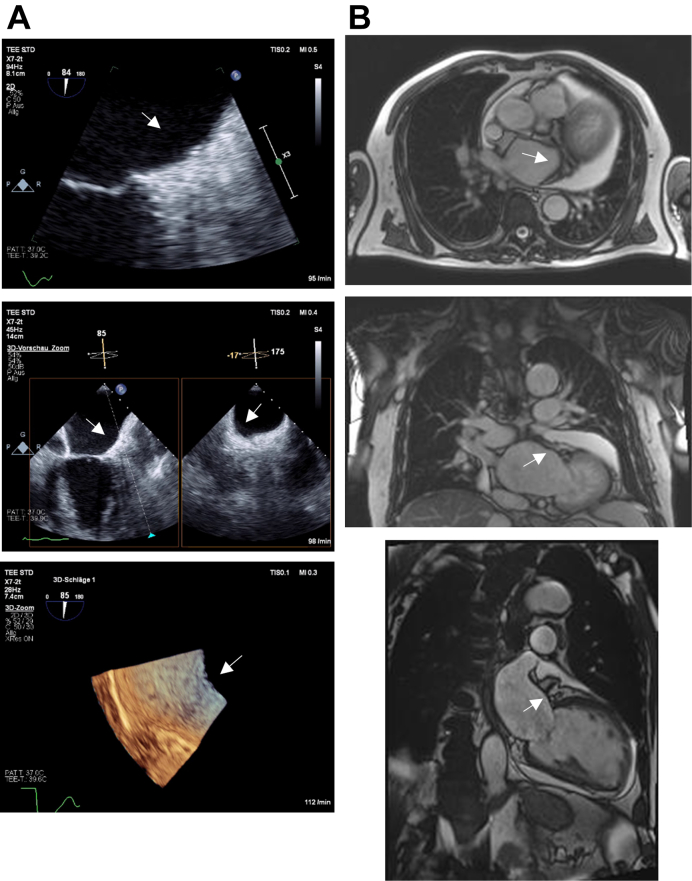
Absence of the Left Atrial Appendage as Demonstrated by Transesophageal Echocardiography and Cardiac Magnetic Resonance Imaging (A) Transesophageal echocardiography images in 3 different views demonstrating the absence of the LAA. (B) Cardiac magnetic resonance imaging confirming the absence of the LAA in 3 distinct views. The arrows mark the region in which the LAA would typically be located. LAA = left atrial appendage.
